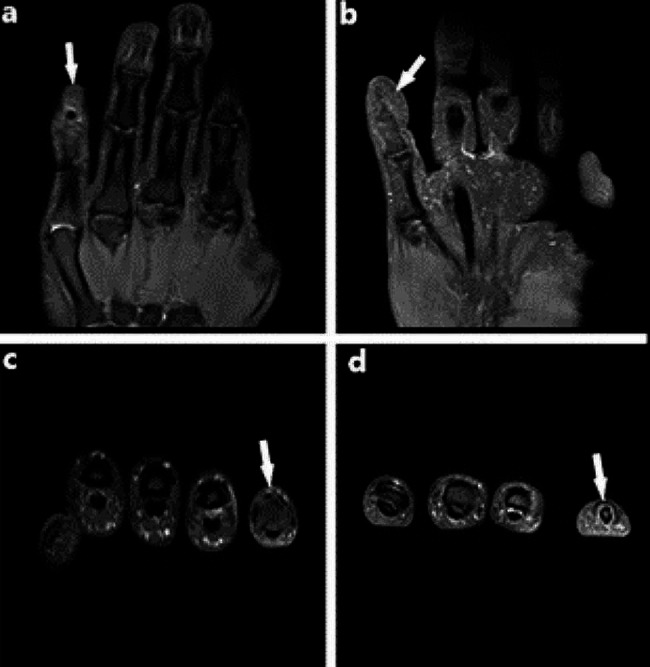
Comparing between MRI before and after the surgery. (a, c) preoperative images, show a foreign body, which did not rupture the extensor tendon, and a poor bone connectivity of the middle and distal phalanges, with patchy (lipid suppression) high signal shadow and unclear boundary at distal phalanx of the little finger(narrows). (b, d) postoperative images, present no foreign body shadow
